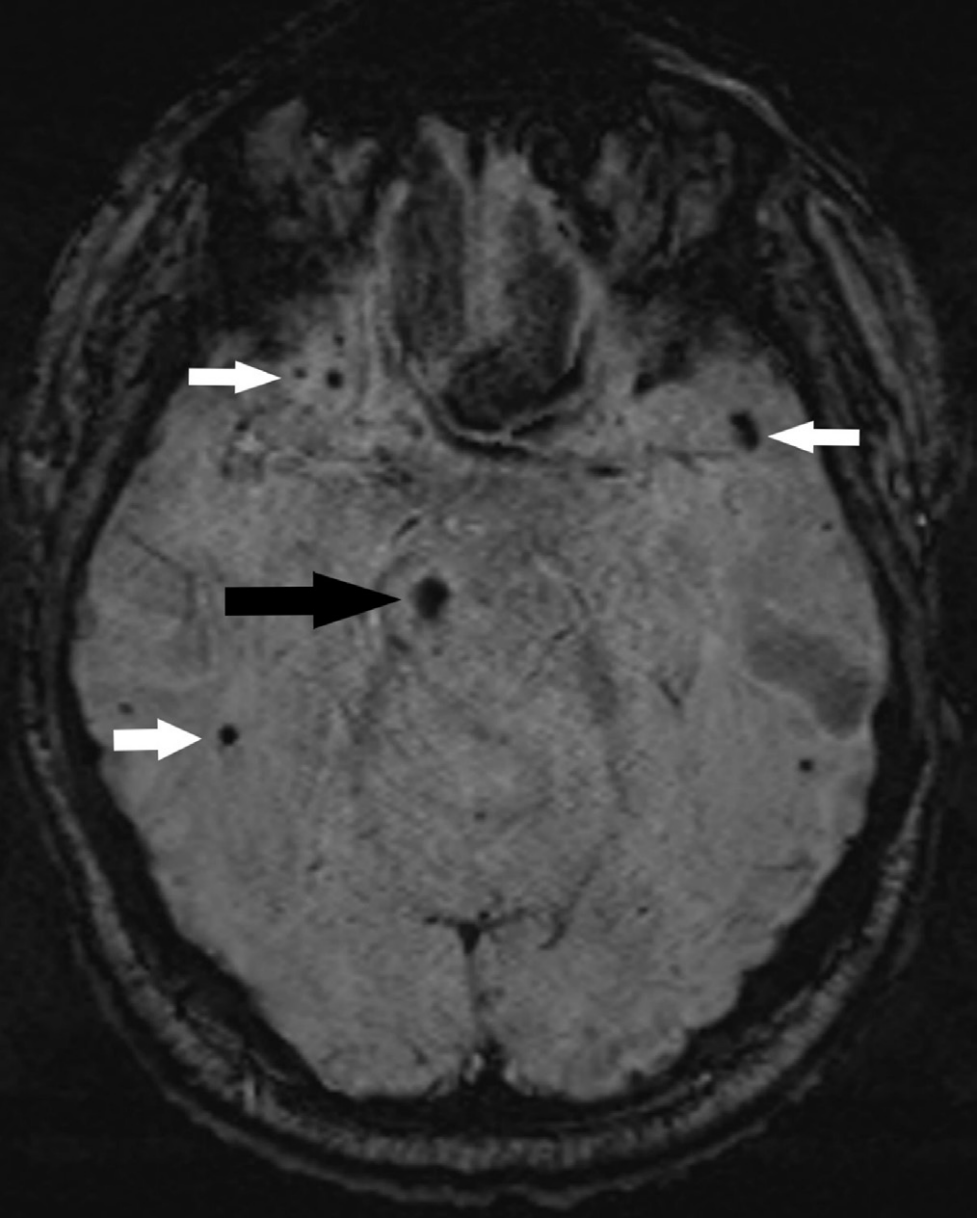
SWI of MRI brain showing haemorrhage within the right substantia nigra (black arrow). In addition to multiple micro-haemorrhages within bilateral frontal and temporal lobes (white arrows).
